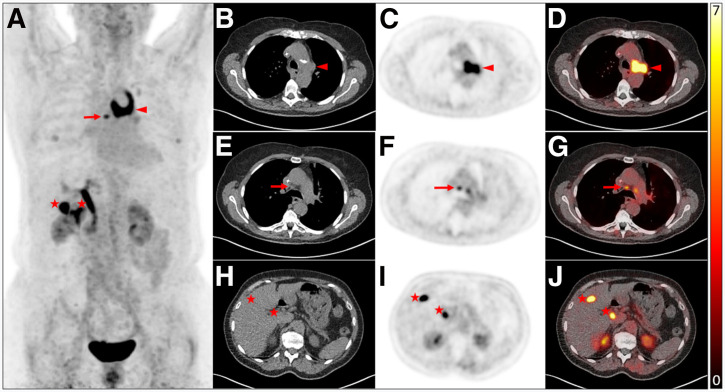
[^18^F]AlF-FAPI-74 PET/CT of 72-y-old woman undergoing primary staging for LC. (A) Maximum-intensity projection image shows intense uptake in central/hilar mass and precarinal lymph node (red arrows), alongside physiologic uptake in the gallbladder, biliary tract (asterisks), kidneys, and bladder. Central mass was rated FAP-RADS 5 by all readers attributable to intense uptake and typical CT morphology (B–D). (E–G) Precarinal lymph node was classified as FAP-RADS 3B by experienced readers; inexperienced readers rated it as FAP-RADS 5 and 3A because of uptake near threshold and minor PET/CT mismatch, respectively. (H–J) Benign uptake in biliary tract and urinary system was consistently rated FAP-RADS 1.
